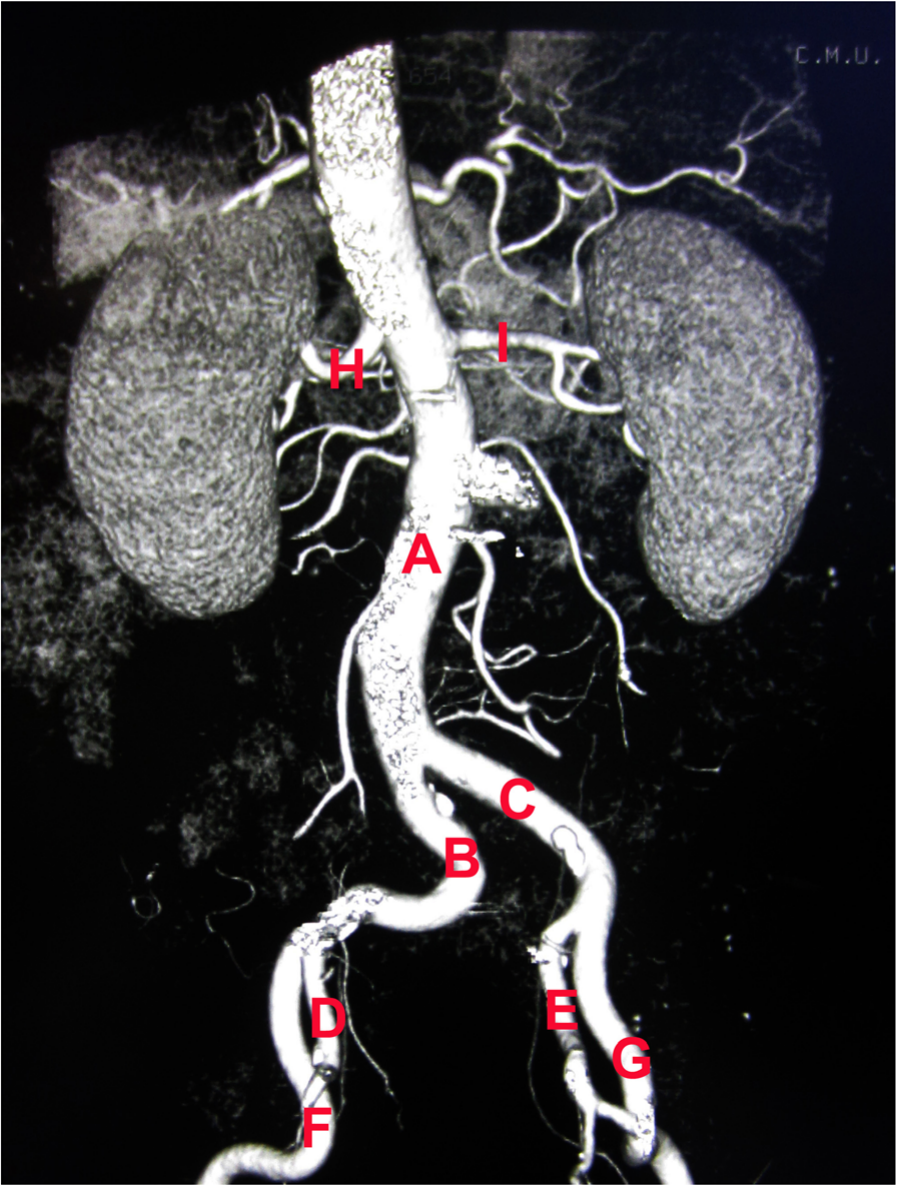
Abdominal aortic CT. (A) Abdominal aorta. (B) Right common iliac artery. (C) Left common iliac artery. (D) Right internal iliac artery. (E) Left internal iliac artery. (F) Right external iliac artery. (G) Left external iliac artery. (H) Right renal artery. (I) Left renal artery.
